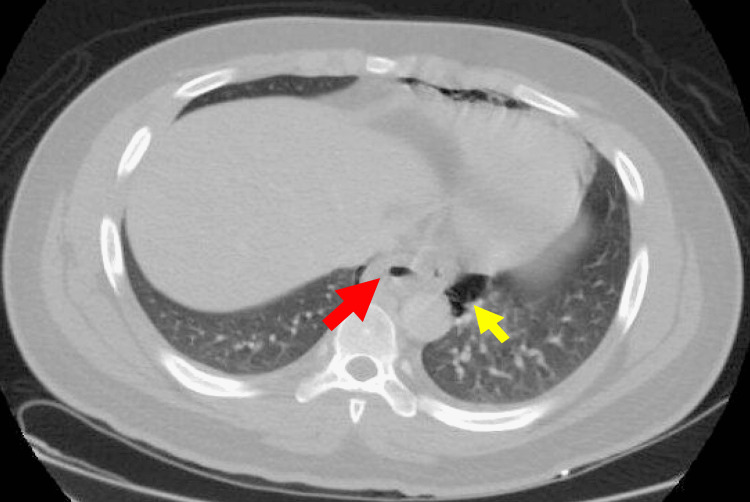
Transverse-view computed tomography of the chest in abdomen window Red arrow demonstrates thickening of the distal esophagus with perforation consistent with Boerhaave syndrome. Yellow arrow demonstrates pneumomediastinum secondary to esophageal perforation.
